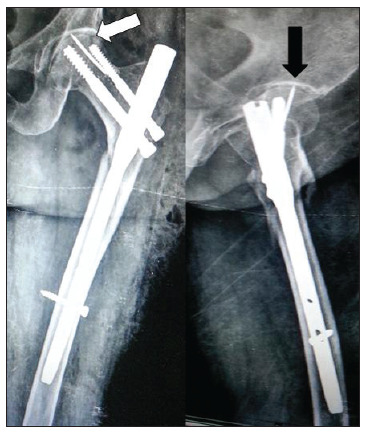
Postoperative radiographs produced after the primary surgery in anteroposterior view (white arrow) and lateral view (black arrow), showing broken guidewire in hip joint and short femoral nail.
